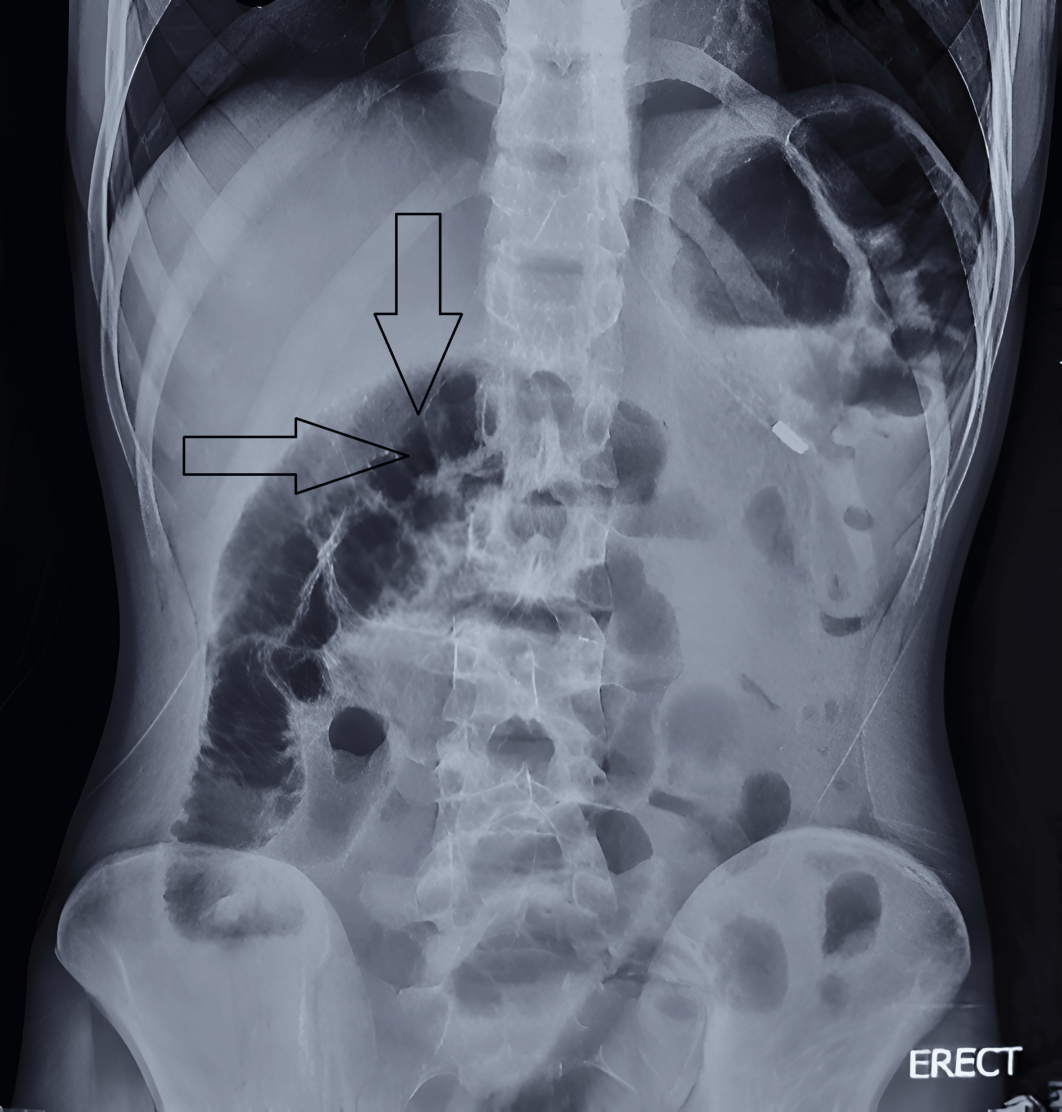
Erect abdominal X-ray showing multiple dilated bowel loops Arrows indicate multiple dilated bowel loops.
